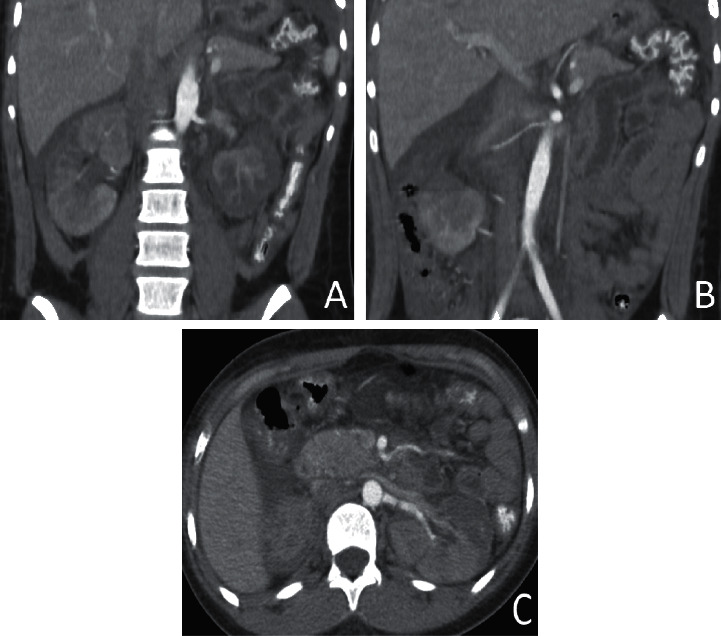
CT scan performed on day 7. (a) Coronal view, arterial phase; renal arteries are both clearly identified, without dissection or thrombosis. (b) Coronal view, arterial phase, 3 right renal arteries, anatomical variant. (c) Axial view, probable unilateral artefact without any flow interruption.
